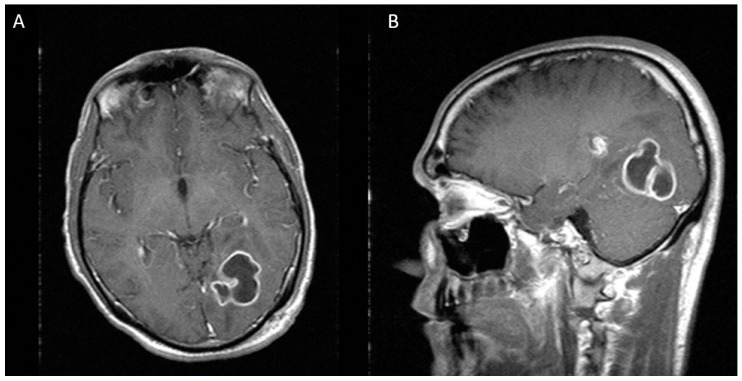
Two weeks after first stereotactic needle aspiration, MRI demonstrates an increase in the abscess size, now measuring 36 × 33 × 40 mm. (A) Axial view (B) Sagittal view.
